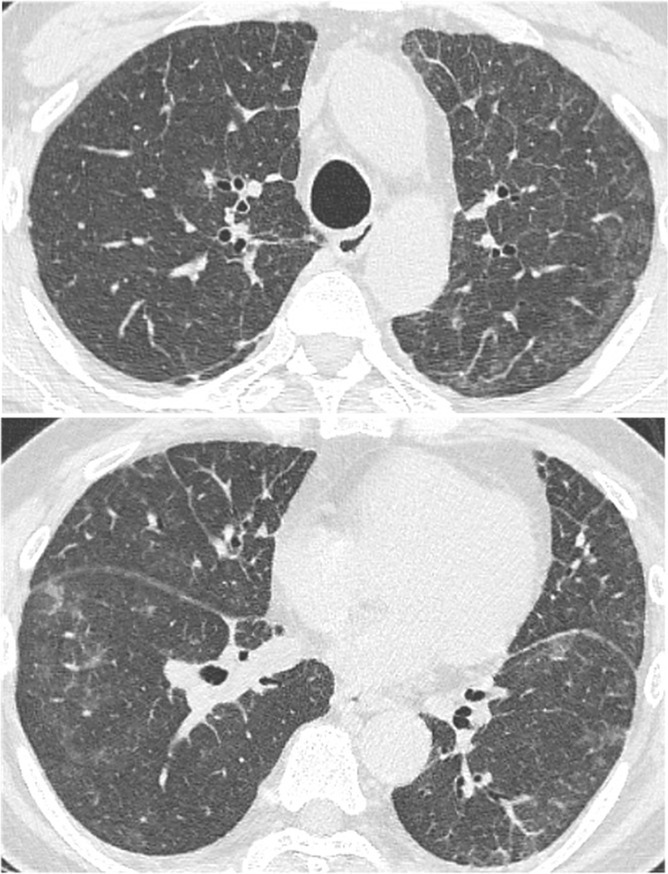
High‐resolution computed tomography of both lungs showing diffuse ground‐glass opacities and thickened interlobular septa in the peripheral regions of both lungs.
